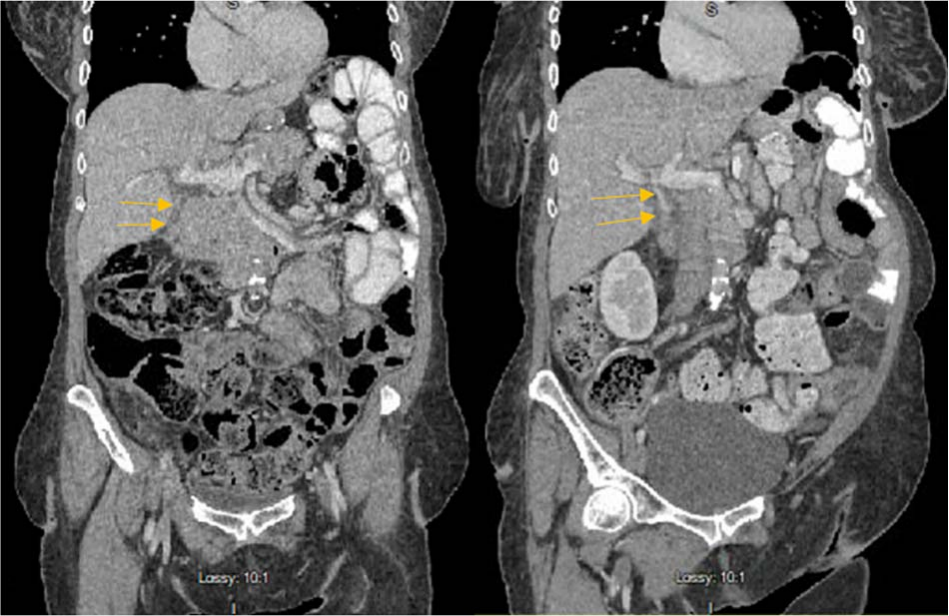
Hyperdense material (arrows) found in the CBD on the post-HIDA CT (right) that was not previously seen on the pre-HIDA CT on admission (left).
